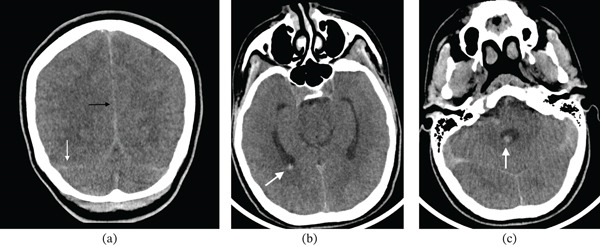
Noncontrast CT of the brain (axial images). (a) Acute subarachnoid haemorrhage along the falx cerebri (black arrow) and tentorium cerebelli (white arrow). (b) Acute intraventricular haemorrhage in the atrium of the right lateral ventricle (white arrow). (c) Acute intraventricular haemorrhage in the fourth ventricle (white arrow).
